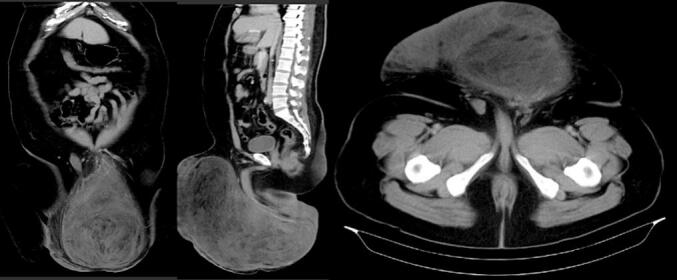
Abdomen-Pelvis CT-Scan with Contrast.
